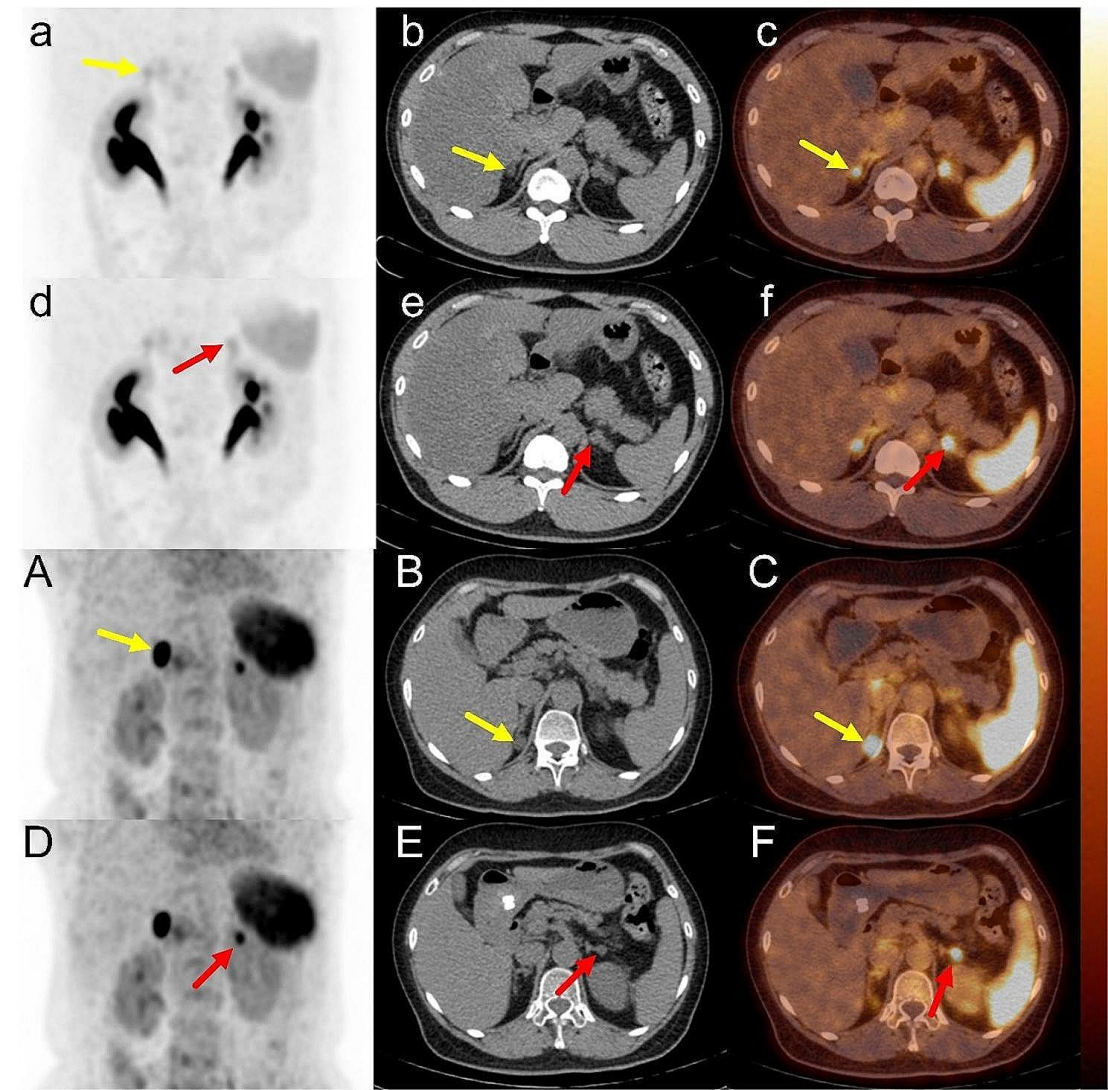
The 10-min ^68^Ga-pentixafor PET lateralization result (a-f) of patient 18 showed BPA, and AVS indicated UPA (right, LI = 18.42). a-c: right adrenal gland, SUVmax = 4.90 (yellow arrow); d-f: left adrenal gland, SUVmax = 4.87 (red arrow); CON = 1.01. 10 min ^68^Ga-pentixafor PET lateralization result (A-F) of patient 6 showed UPA (right), and AVS indicated BPA (LI = 1.73). A-C: right adrenal gland, SUVmax = 20.20 (yellow arrow); D-F: left adrenal gland, SUVmax = 9.55 (red arrow); and CON = 2.12.
